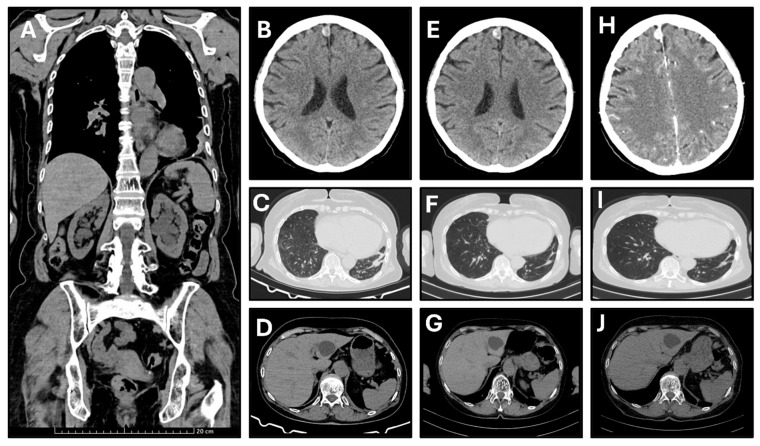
(A). Baseline coronal CT scan obtained on 31 October 2024. (B–D). First restaging axial CT scans performed on 31 January 2025, after 3 months of treatment. (E–G). Second restaging axial CT scans performed on 24 September 2025, after 9 months of treatment. (H–J). Third restaging axial CT scans performed on 19 January 2026, after 12 months of treatment.
